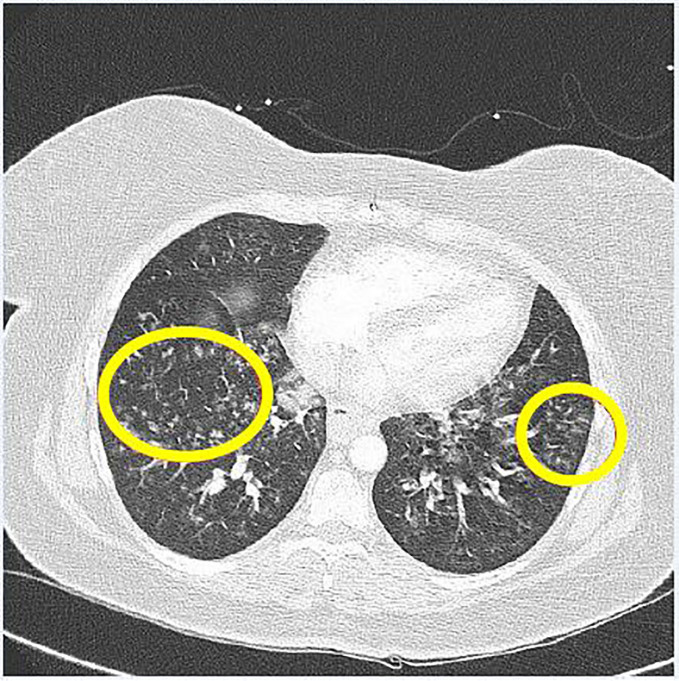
Computed tomography of the lower lung and heart with demonstration of nodular infiltrates at the lung bases, which are circled.
